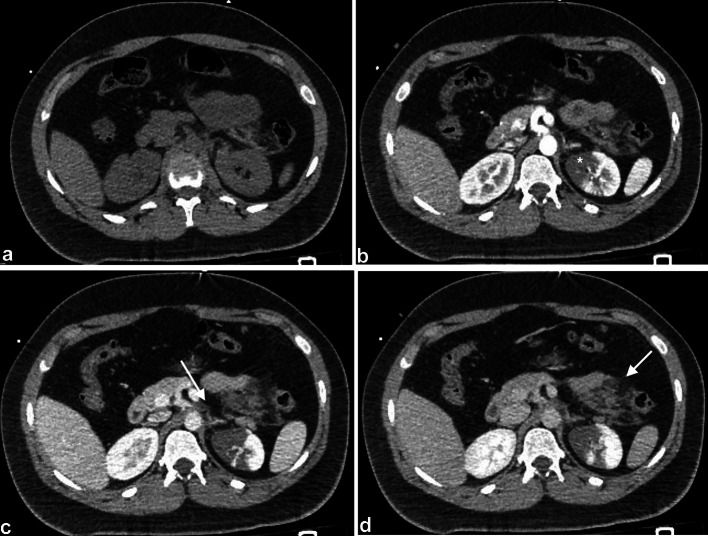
CT axial without contrast (A), during the arterial phase (B) nephrographic phase (C), excretory phase (D). In the upper pole of the left kidney appeared, a well-demarcated area of reduced/absent contrast enhancement in all phases was detected (B white asterisk). Non perirenal fluid or subcapsular hematoma were present there was a mildly fat stranding in the para-aortic region (C ached arrow). Moreover, CT showed mesenteric fat standing in left hypochondriac region suggestive of mesenteric hematoma without active bleeding or bowel perforation (white arrow)
